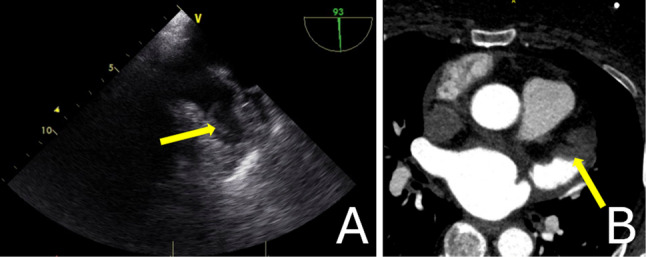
TEE and cardiac CT images from a 62-year-old woman with AF and a LAA thrombus. A TEE demonstrated a thrombus (arrows) with SEC. B CT demonstrated an oval-shaped filling defect in the LAA (arrows) suggestive of thrombus
